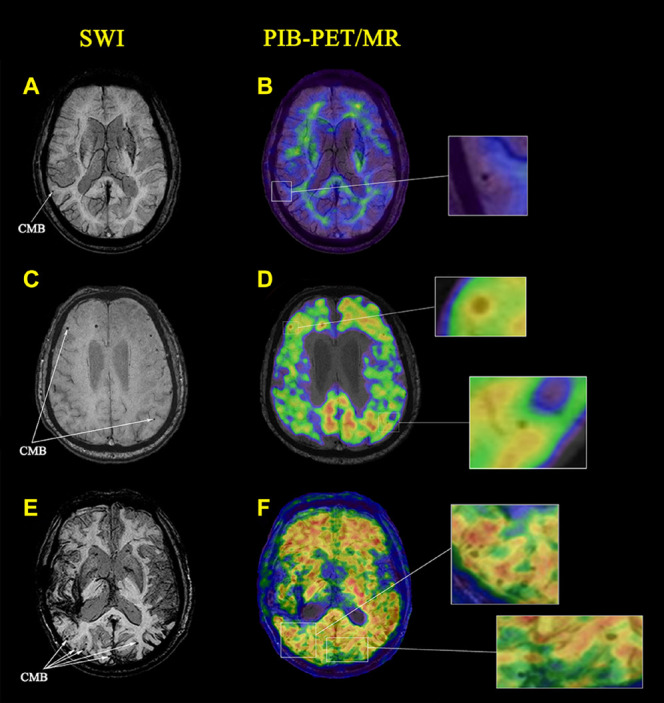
Cerebral microbleeds (CMBs) identified on images from magnetic resonance imaging (MRI) and PIB PET in a representative cognitively normal healthy control subject (NC) and patients with Alzheimer’s disease (AD) and CAA. (A) An axial susceptibility-weighted imaging (SWI) MRI scan from a 70-year-old woman with hypertension. A CMB is present in the right temporal lobe (white arrows). (B) A PIB-PET scan shows no lobar amyloid deposition (amyloid-negative) with only non-specific white matter deposition. (C) An axial SWI MRI scan from a 64-year-old man with long-standing hypertension. CMBs are present in the right frontal and left temporal lobes (white arrows). (D) A PIB-PET scan shows increased amyloid deposition in the right frontal lobe with no amyloid deposition in the left temporal lobe. (E) An axial SWI MRI scan from a 70-year-old woman without hypertension. CMBs are present in the bilateral occipital and temporal lobes (white arrows). (F) A PIB-PET scan shows widespread cortical amyloid deposition.
